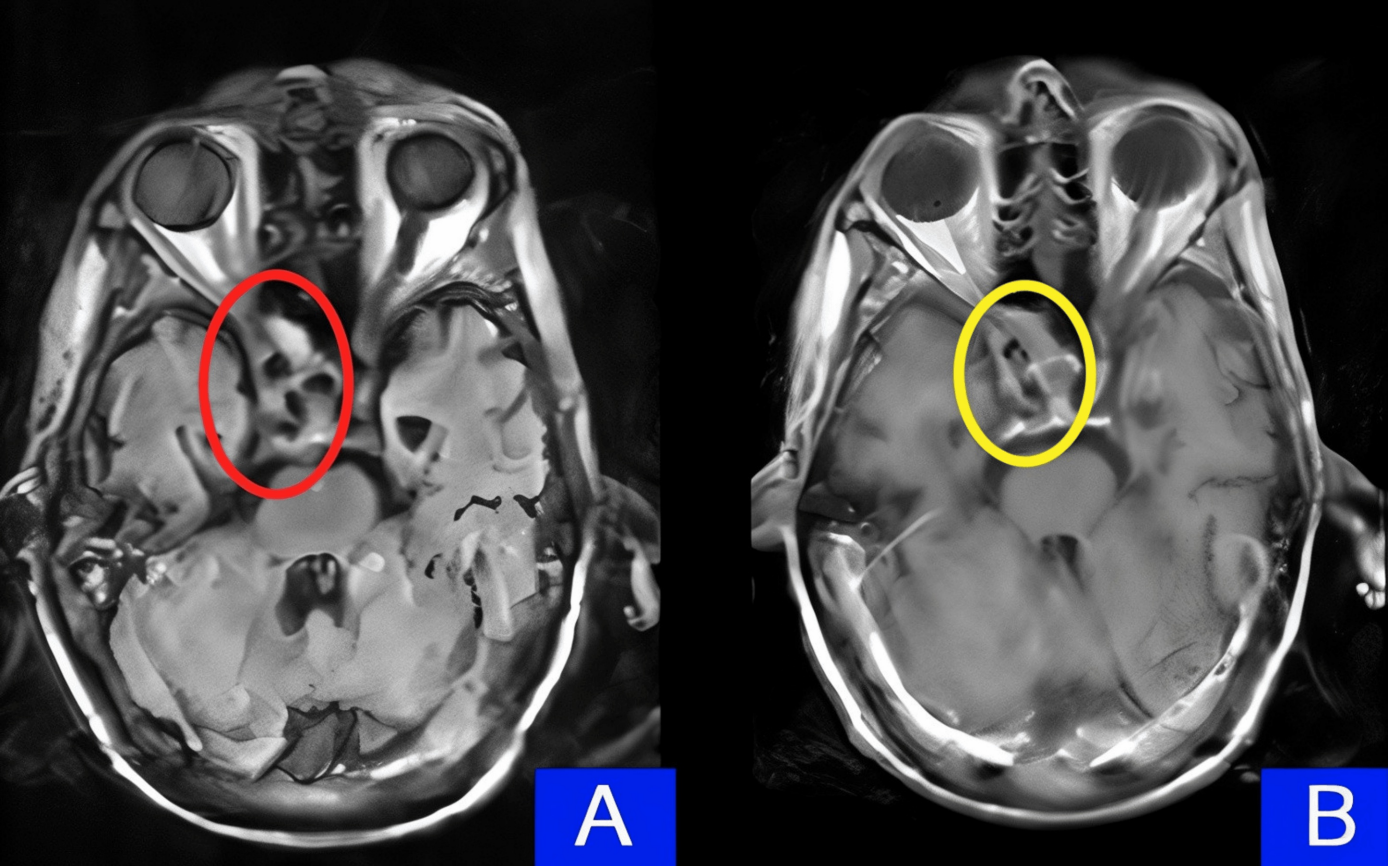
MRI brain: (A) FLAIR sequence showing meningeal signal enhancement in the right anterior cavernous sinus (circled in red). (B) MRI brain without contrast showing pachymeningitis (circled in yellow)
